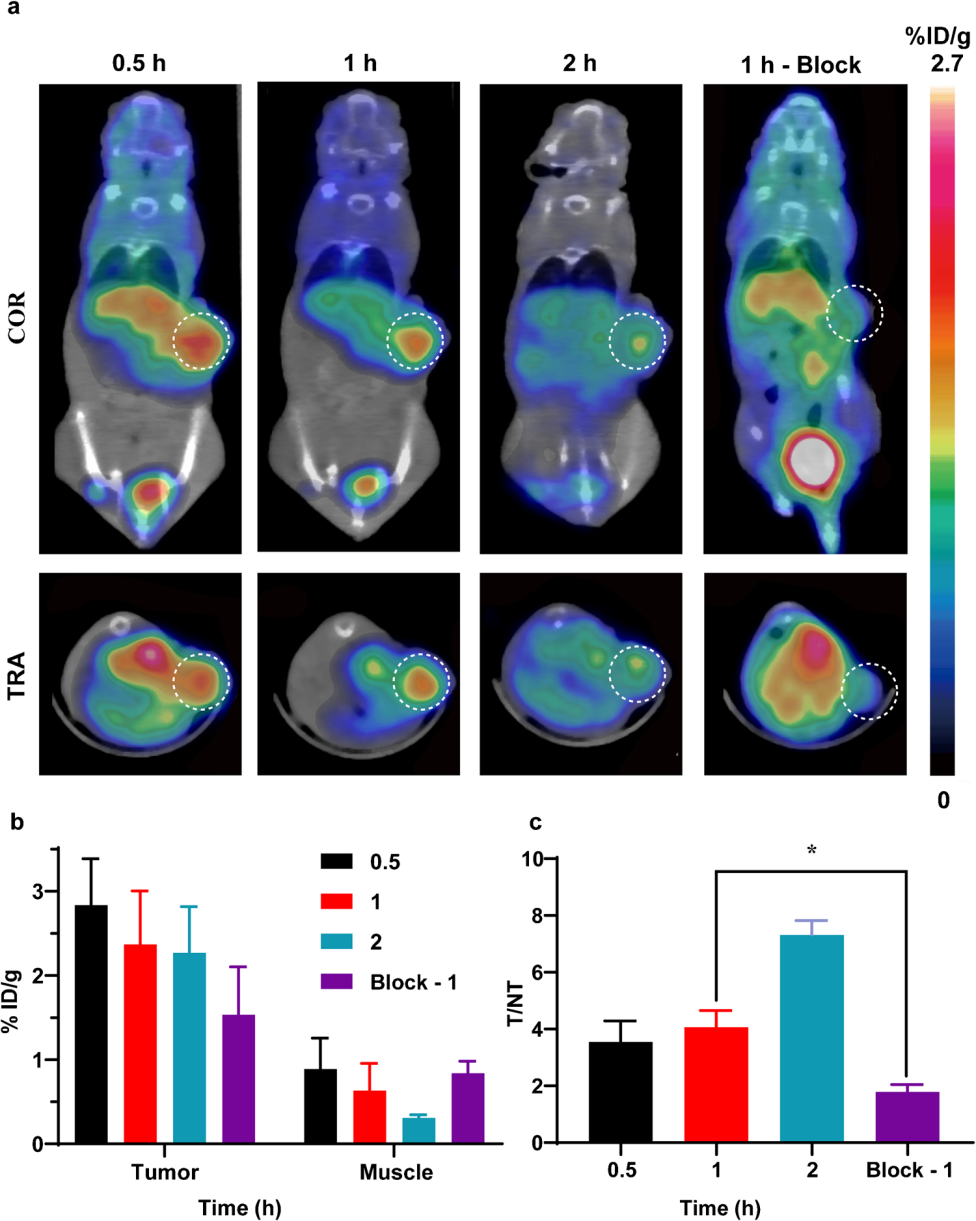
PET imaging of ^68^Ga-DOTA-Olaparib. (a) MicroPET/CT images of.^68^Ga-DOTA-Olaparib in SK-OV-3 models at 0.5, 1, and 2 h after administration. (b) Quantification of the regions of interest (ROIs) in SK-OV-3 models expressed as %ID/g. (c) T/M (tumor-to-muscle) ratios (P* = 0.0238 < 0.05, *n* = 3)
